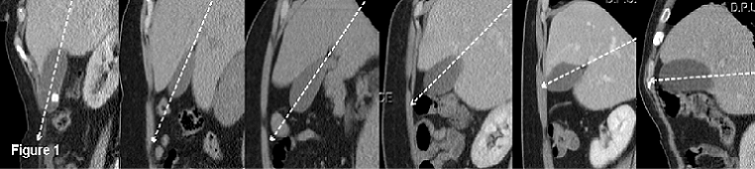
Gallbladder angle varies considerably from person to person on sagittal reformatted CT images
